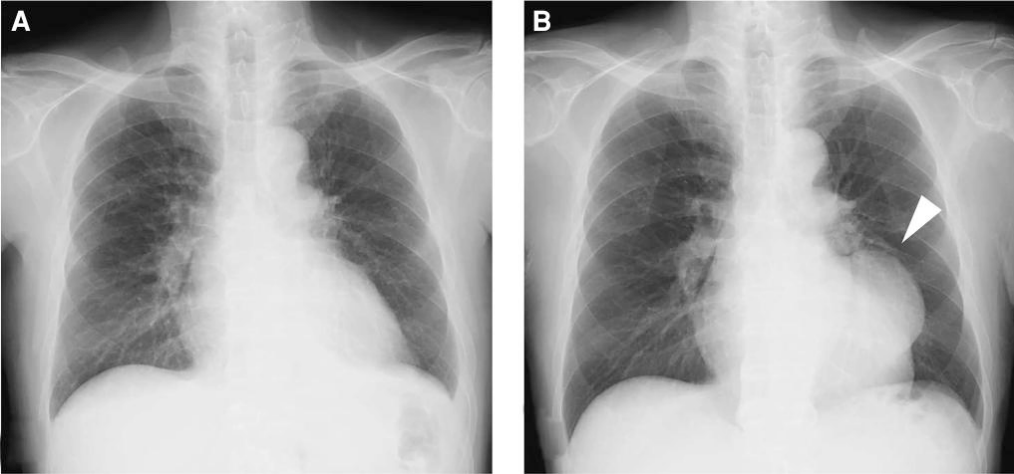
Chest radiographs. (*A*) At the first presentation. (*B*) At discharge after the second intervention showing a mass suspicious for left ventricular pseudoaneurysm (arrow).
